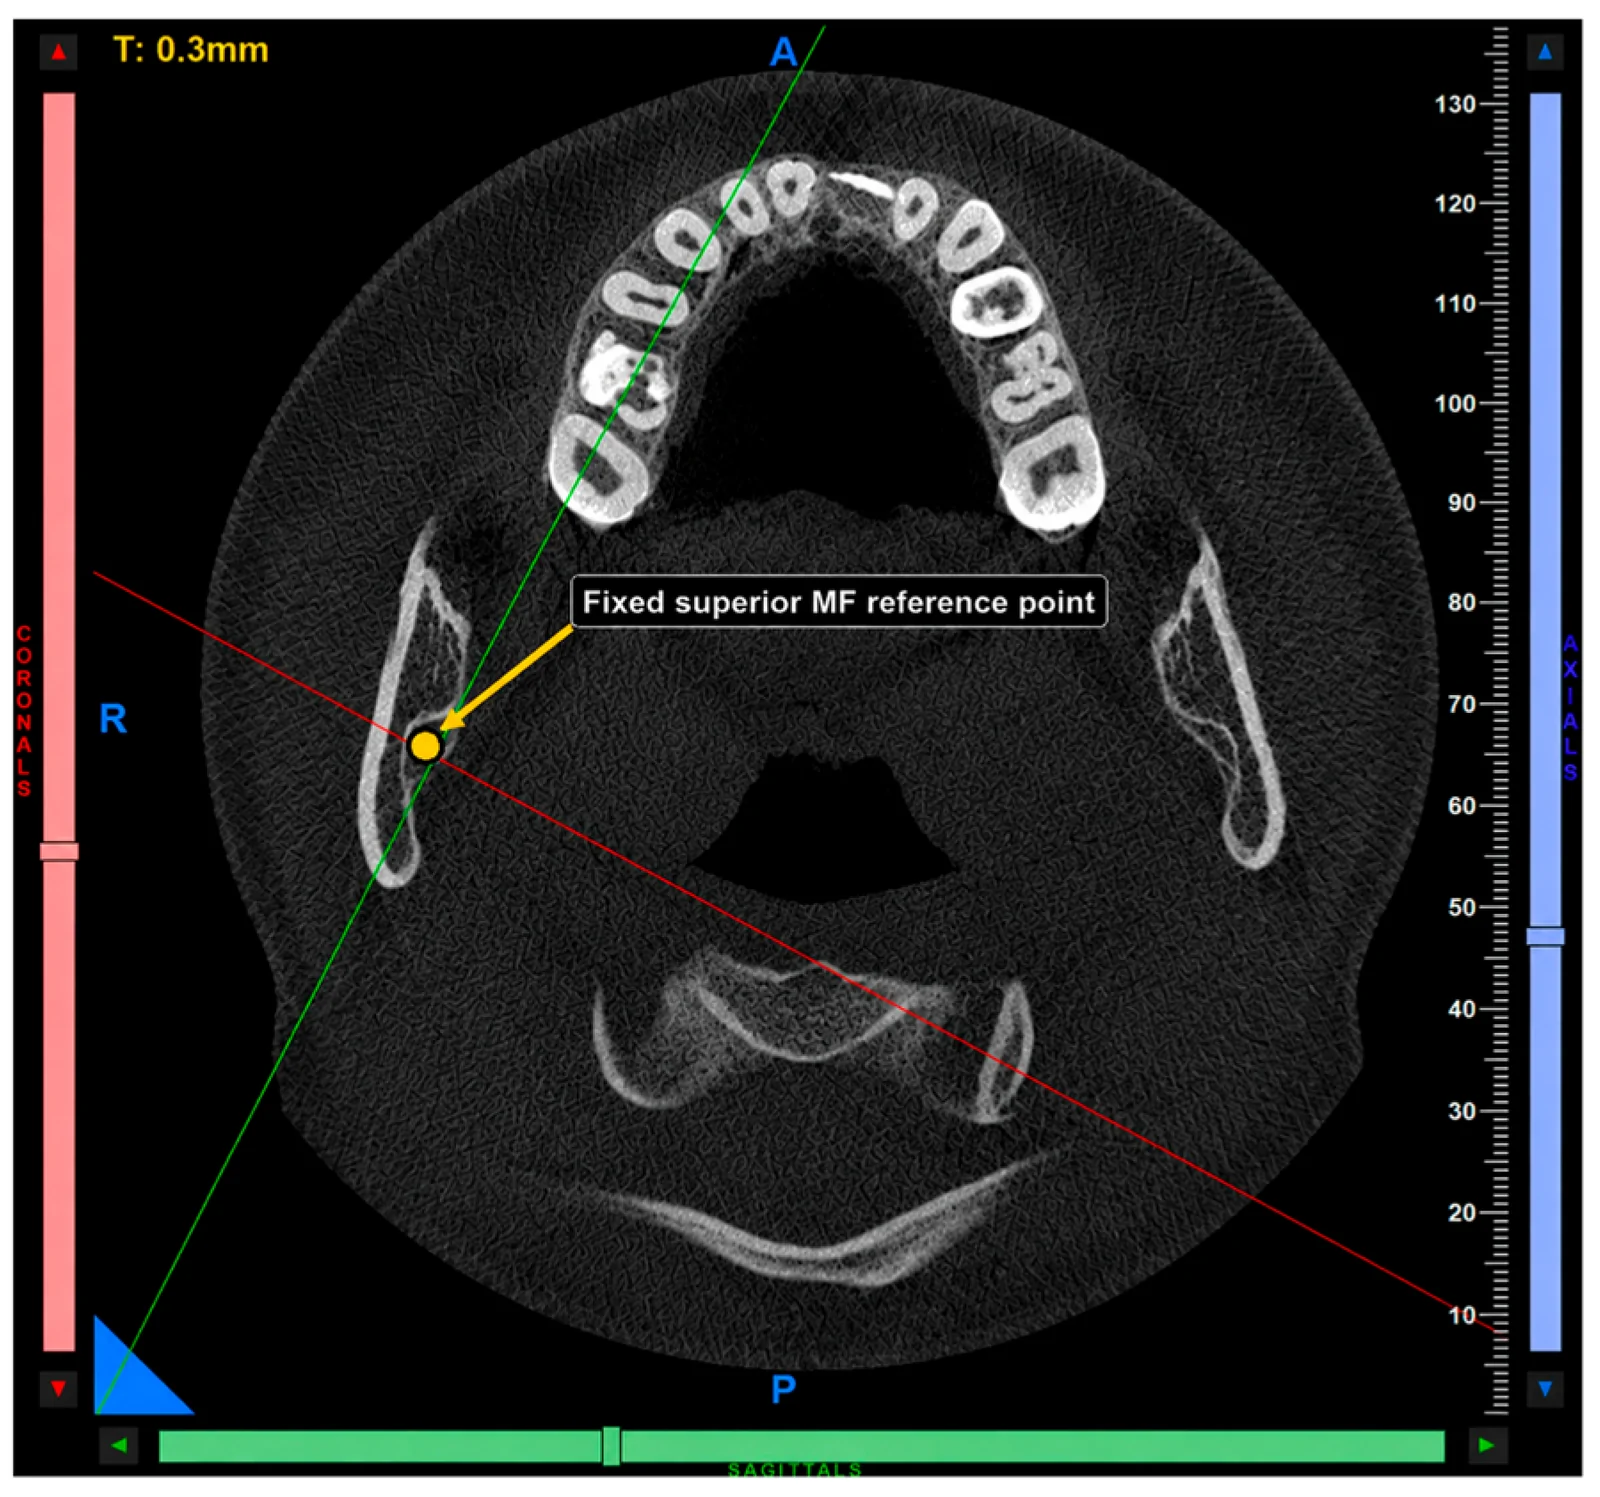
Axial CBCT image showing the fixed reference point on the superior border of the mandibular foramen (MF). This reference point was maintained throughout the multiplanar reconstruction process for the M1, M2, and M3 measurements.
